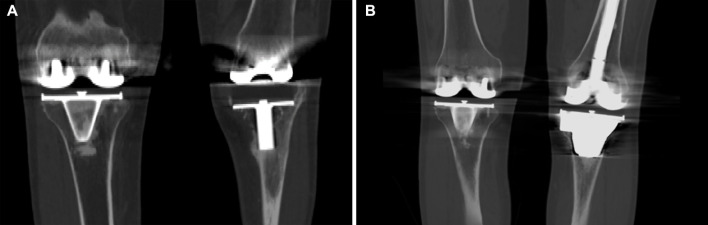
CT images of the representative case showed that the tibial bone defect was effectively reconstructed by the porous tantalum cone. (A) Preoperative image; (B) postoperative image at 3 years after the operation.
